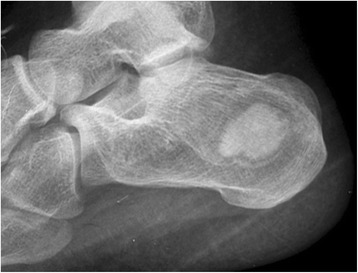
Lateral radiograph of the left calcaneum. An expansive, partially osteolytic lesion is displayed, with well-defined margins, and an eccentric calcified matrix
